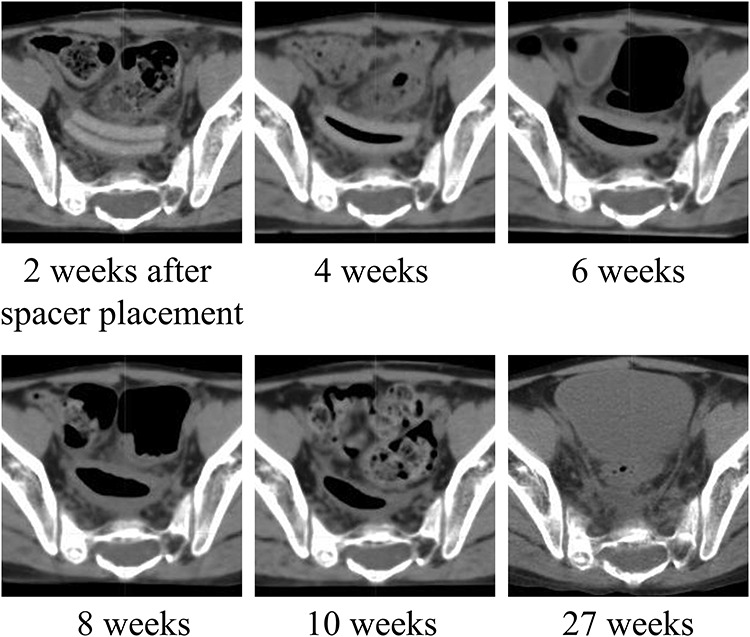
Serial CT images showing morphological changes in spacer reduction over time.
